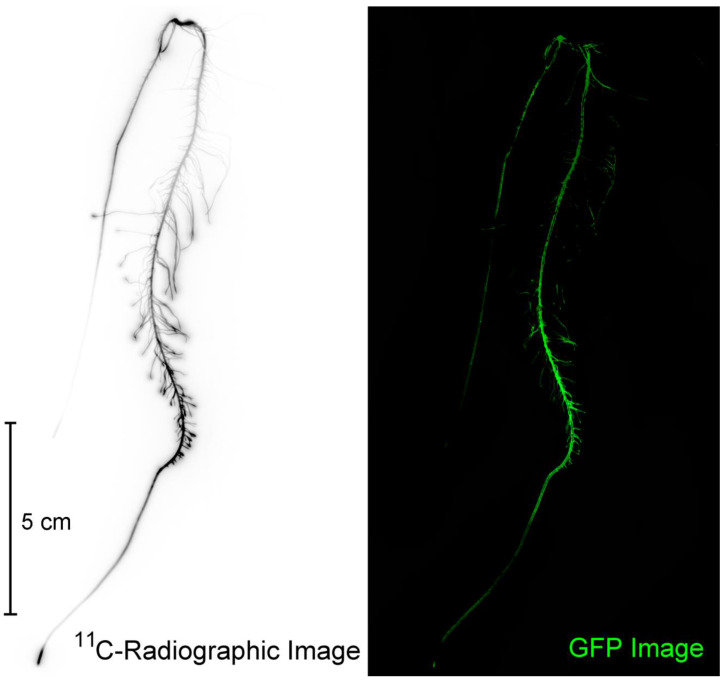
A comparison of the same root subjected to ^11^C-radiographic imaging and GFP imaging on the Typhoon 9000. Using nuclear-based and optical-based imaging, we were able to identify regions of high ^11^C-activity and bacteria colonization.
